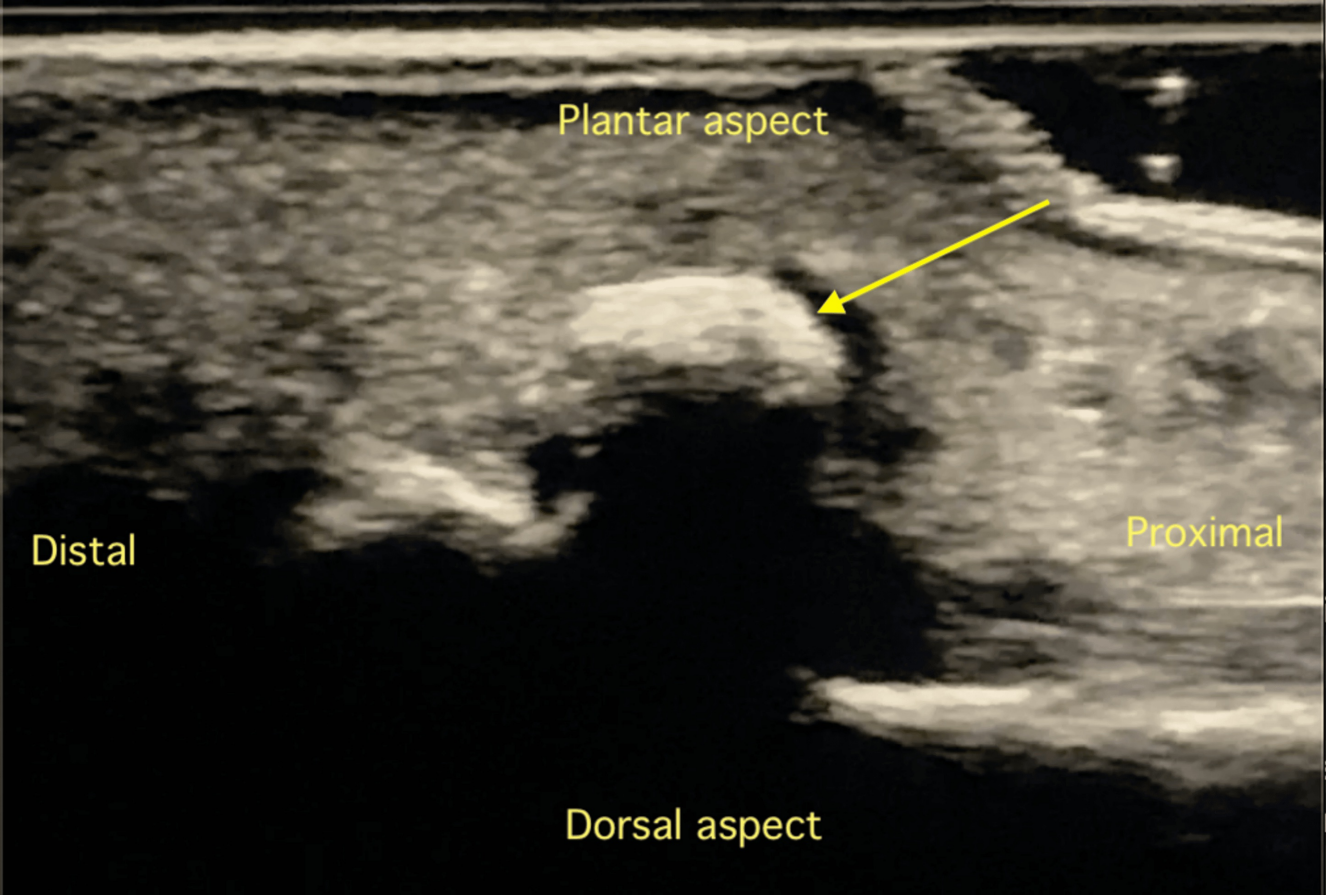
Ultrasound image using a linear transducer of extra-articular calcification (arrow) at the plantar aspect of distal interphalangeal joint
